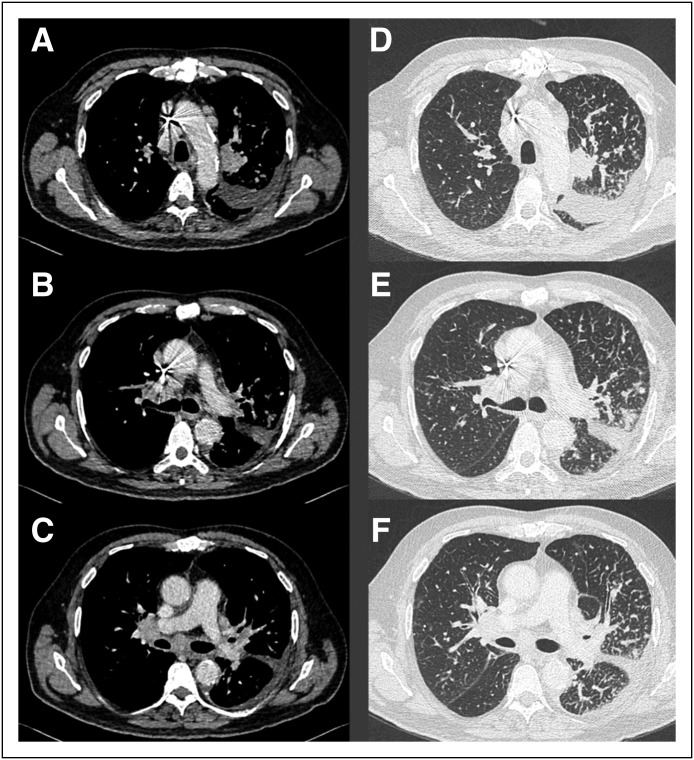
Computed tomography scan showing progressive disease characterized by
